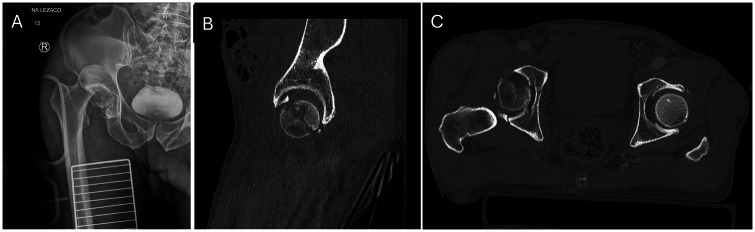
Anteroposterior preoperative radiograph of the pelvis displaying dislocation of the right hip joint after the closed reduction attempt (A). Preoperative CT image with sagittal (B) and coronal view (C), demonstrating femoral head fracture with posterior acetabular wall involvement.
